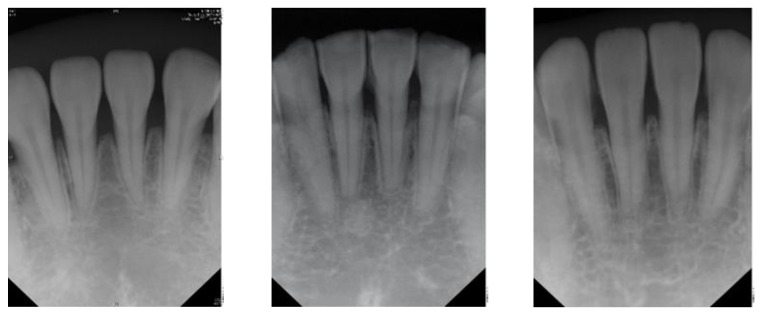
Periapical film database.
